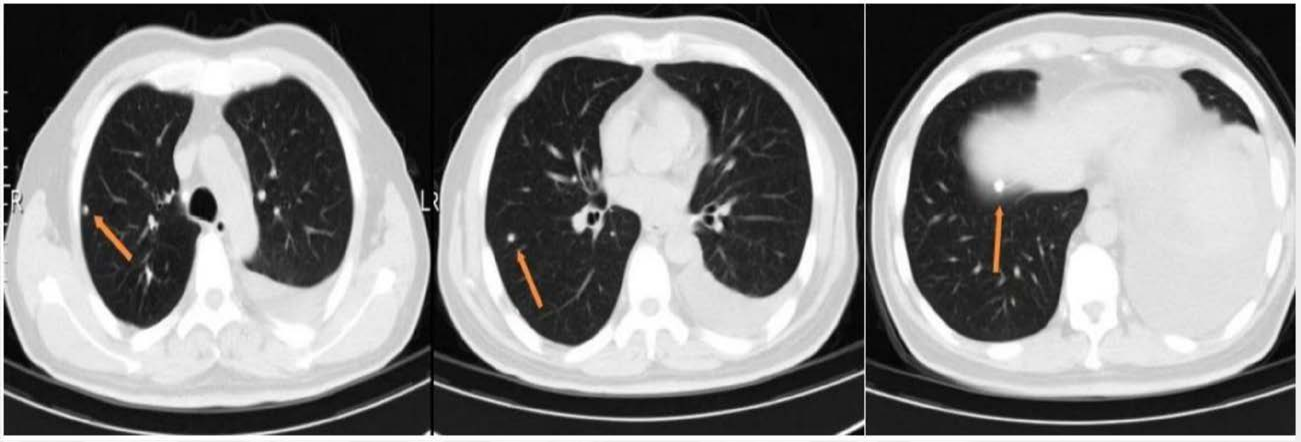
Chest CT showed multiple calcified nodules in the right upper and lower lobes. CT = computed tomography.
